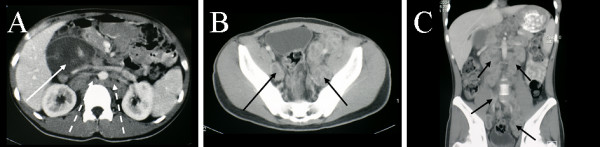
Multiplanar abdominal and pelvic CT, demonstrating extensive lymphadenopathy. A. Large low attenuation portal lymph node (solid arrow) showing peripheral enhancement, and associated para-aortic nodes (dashed arrows). B. Extensive heterogenous attenuation side wall pelvic adenopathy. C. Coronal image, demonstrating extensive low attenuation para-aortic and pelvic lymphadenopathy (arrowed)
